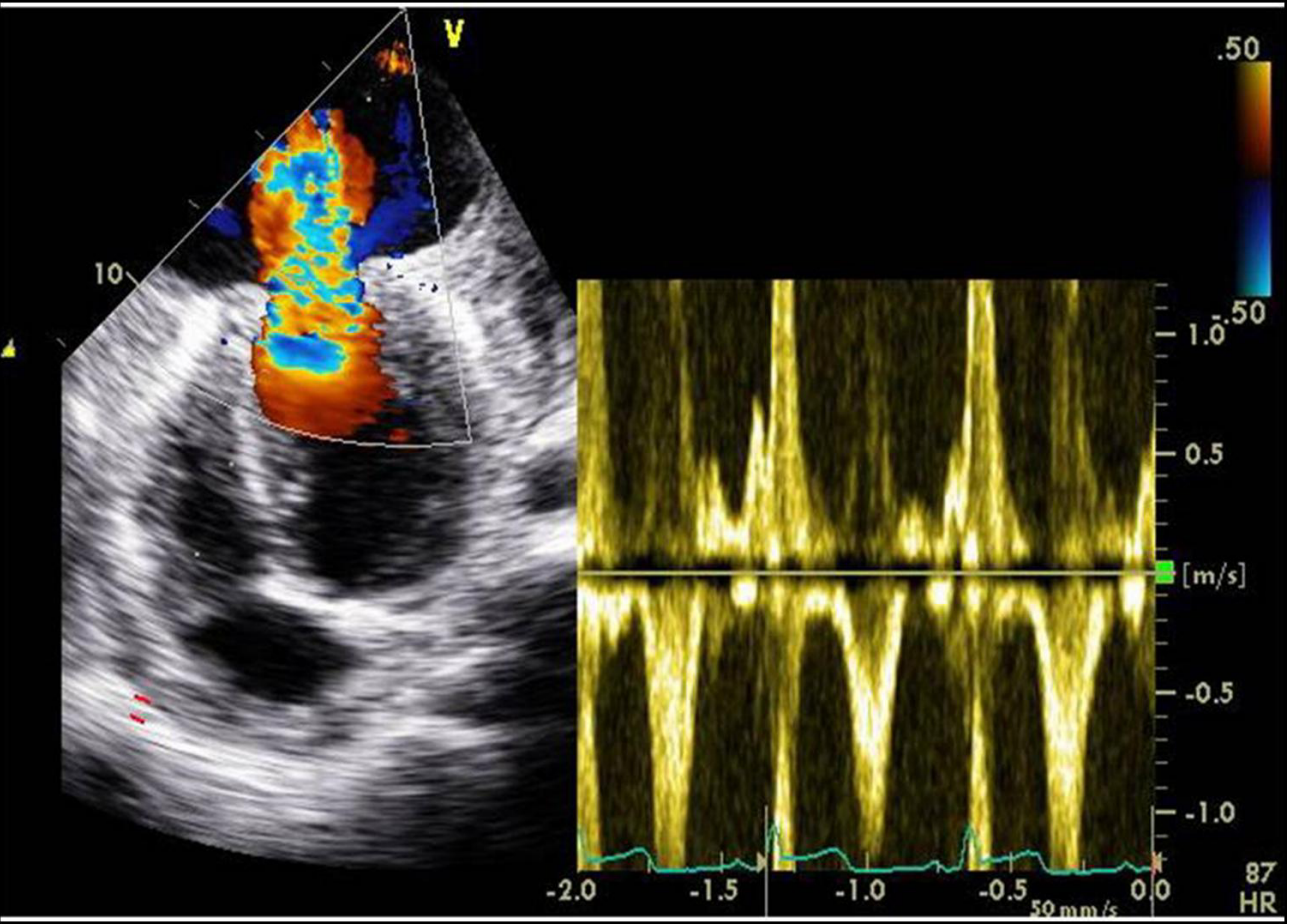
Continuous-wave Doppler of flow across the neck of the pseudoaneurysm. Bidirectional turbulent flow into the pseudoaneurysm in systole and away from the pseudoaneurysm, into the left ventricular cavity, in diastole.
